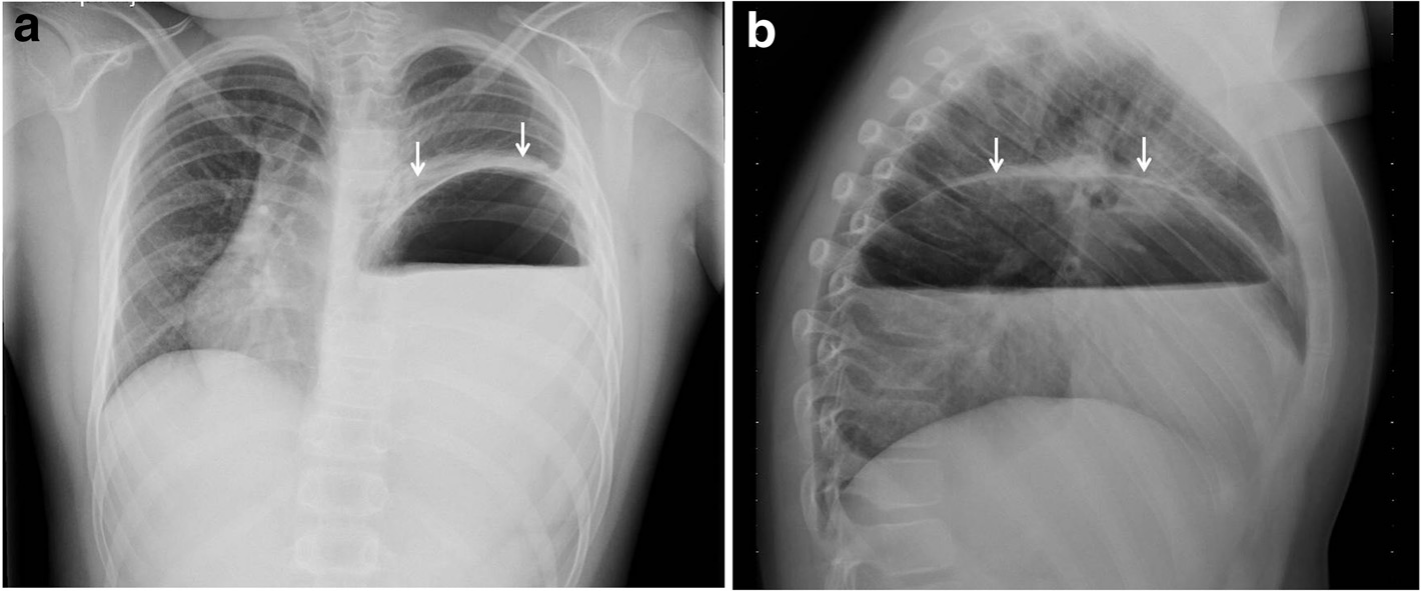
Anteroposterior (a) and lateral (b) chest radiographs with large air-fluid level in left hemithorax. Note superior rim formed by stomach wall and compressed lung (arrows) and mediastinal shift to the right
